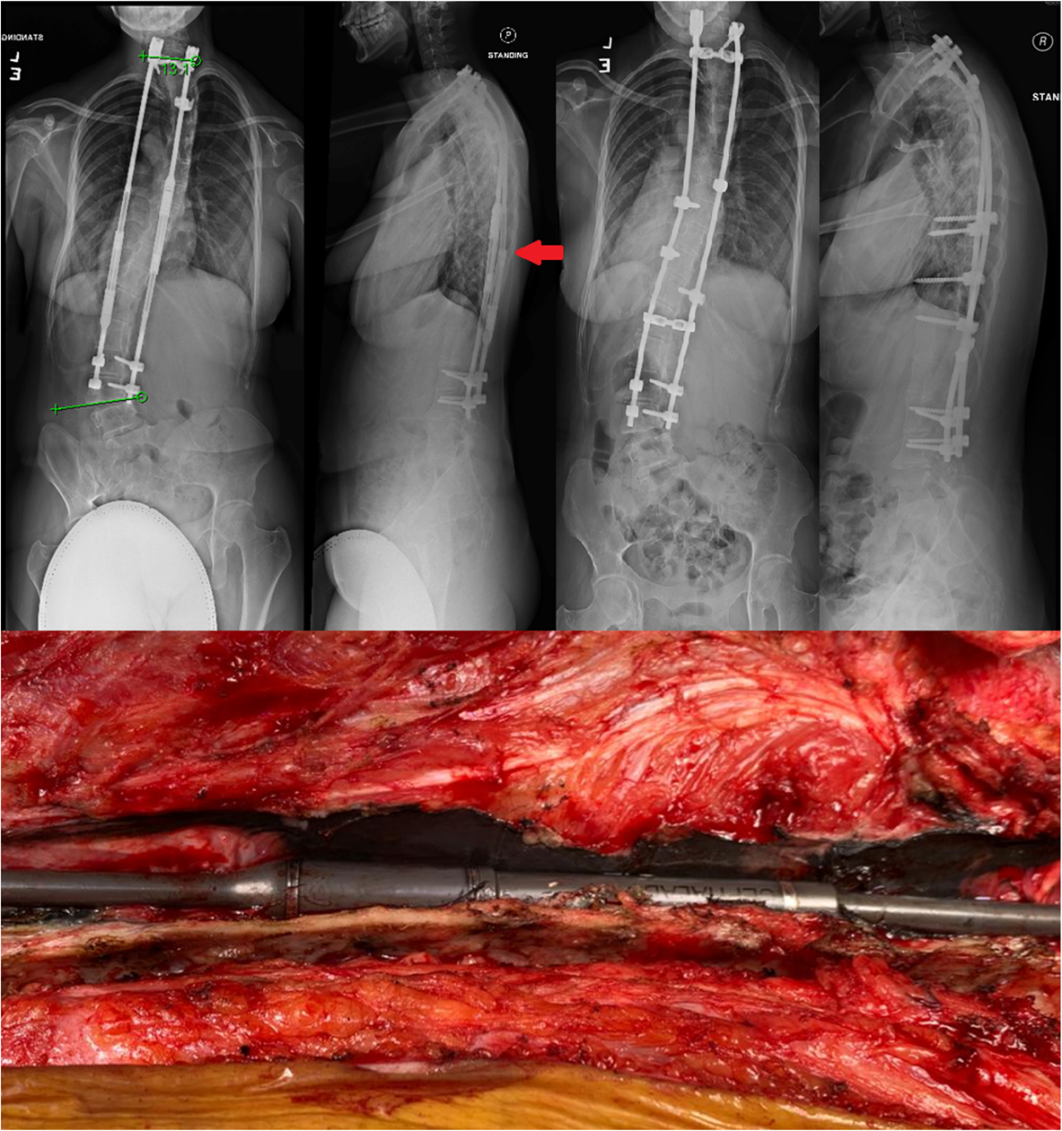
Pre-final surgery posteroanterior and lateral radiographs identified a crooked rod sign and autofusion of the lumbar spine (top left). Final fusion surgery from C7-L4 was performed (top right) leaving a residual tilt at L4-sacrum. Intraoperative photo showed extensive metallosis around the actuator and extendable portion of the rod (bottom)
